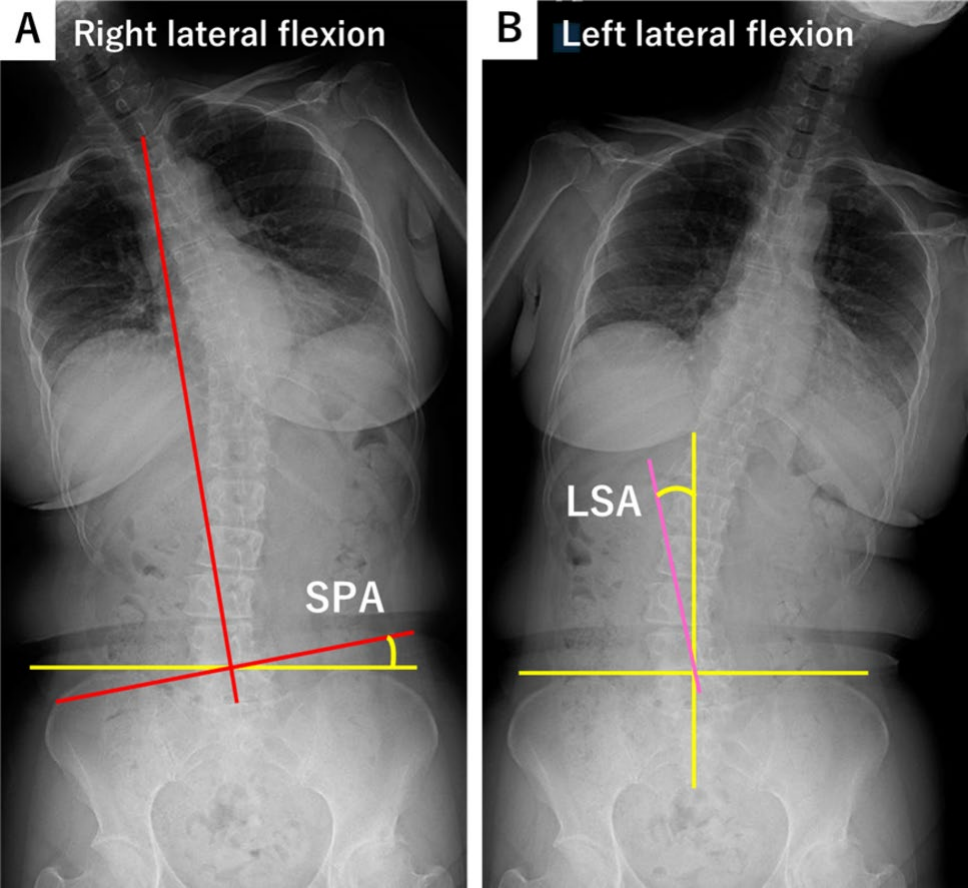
Standing whole-spine anteroposterior radiographs in right lateral bending and left lateral bending positions. Methods for measuring spinopelvic angle (SPA) and lumbosacral angle (LSA) were demonstrated. The SPA is the angle between a line drawn perpendicular to the middle of T1 to S1 (red) and the iliac crest line (yellow). The LSA is the angle between a line drawn through the spinous processes of L4 and L5 (pink) and is perpendicular to the iliac crest line (yellow). ΔSPA was defined as the absolute difference in SPA between maximum right and left lateral flexion positions. Similarly, LSA was defined as the absolute difference in the LSA between the maximum right and left lateral flexion positions. *SPA* spinopelvic angle, *LSA* lumbosacral angle
